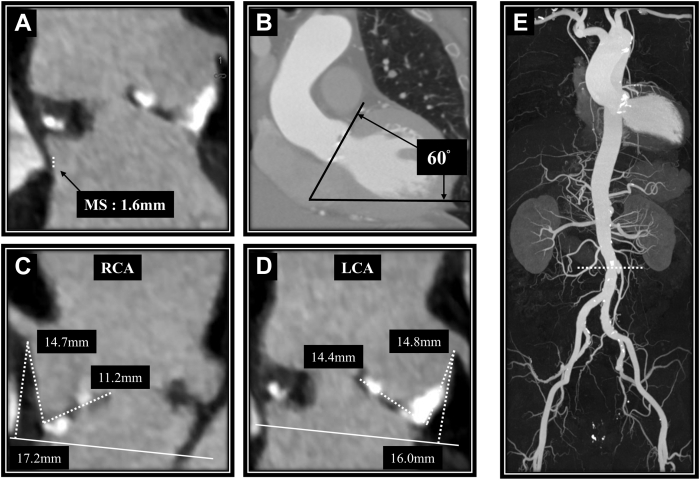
Other Preoperative Computed Tomography Findings (A) Membranous septum (MS). (B) The angle between the aorta and the heart. (C and D) The height of the right coronary artery (RCA) and left coronary artery (LCA). (E) The characteristics of the aorta and lower limb vessels.
